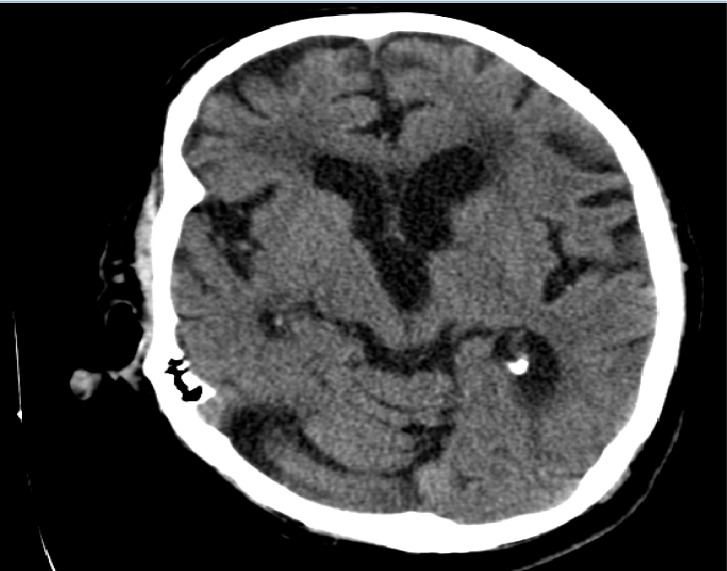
Brain CT scan of the patient at admission
